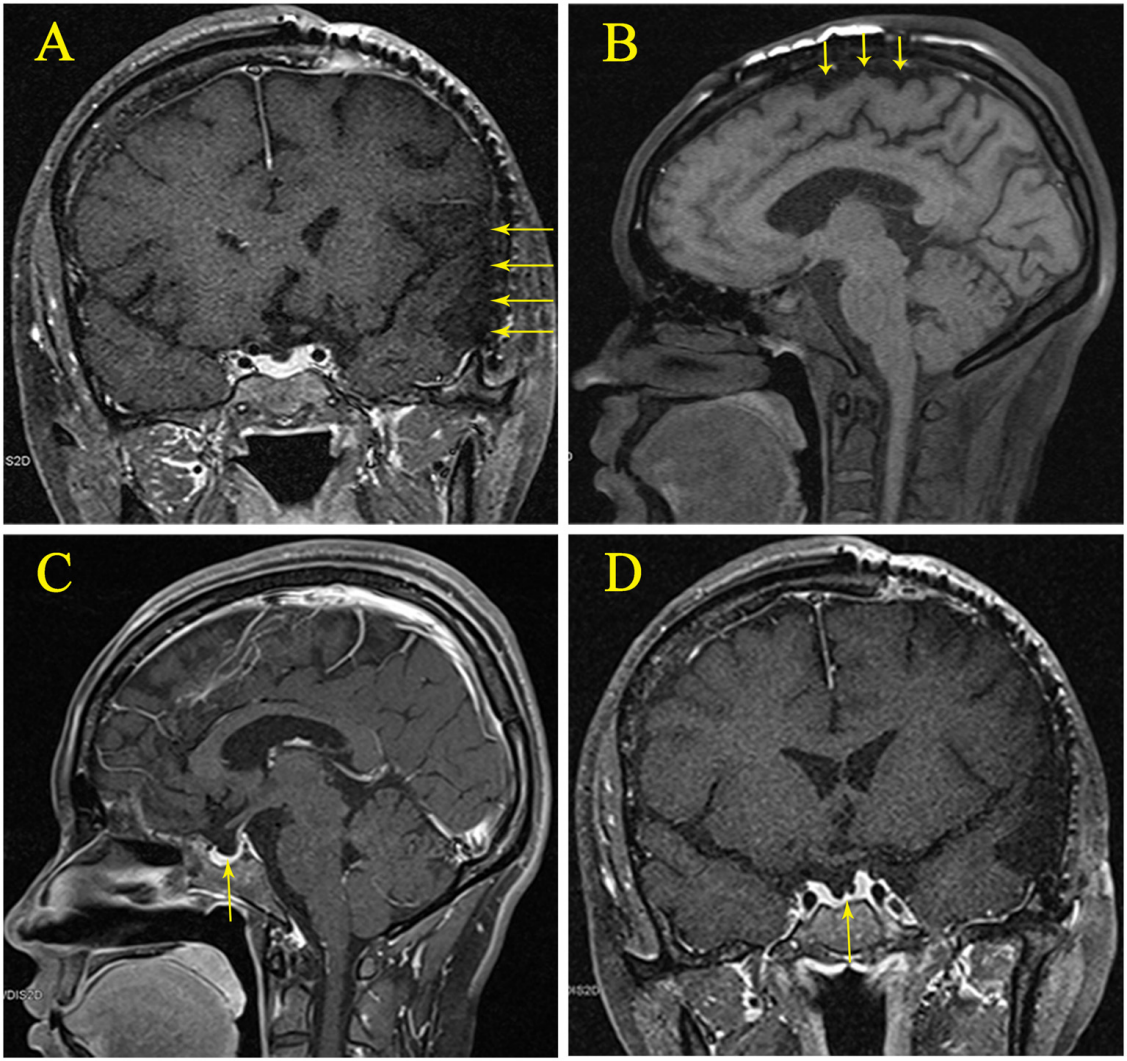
(A) Magnetic resonance imaging (MRI) showing post-traumatic encephalomalacia in the left parietal, temporal, and insular lobes. (B) MRI showing post-traumatic encephalomalacia in the parietal lobe. (C) Sagittal view of an empty sella with the pituitary gland. (D) Coronal view of an empty sella with the pituitary gland.
